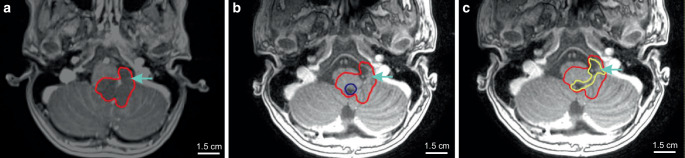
Example of a major/unacceptable deviation; a shows the pre-surgery MRI with the initial tumor volume (*red*); b shows the post-surgery MRI with the GTV_tumorbed_ (*blue*) of the local radiation oncologist, which does not encompass the MRI visible resection cavity/areas with initial tumor contact (initial tumor volume, *red*); c shows the reference GTV_tumorbed_ (*yellow*) and the initial tumor volume (*red*). The *green arrow* marks the region of deviation. *MRI* magnetic resonance imaging, *GTV* gross tumor volume
